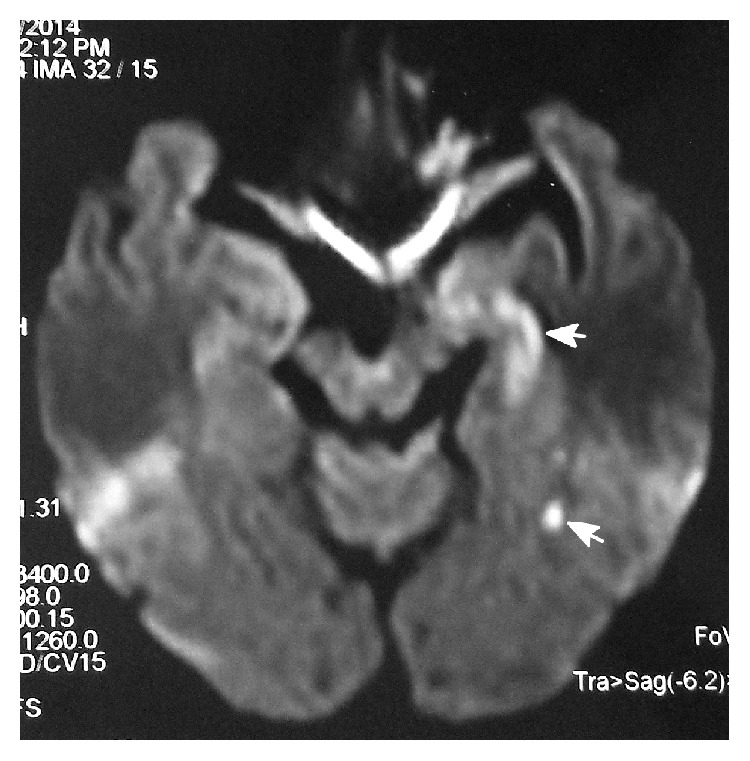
DWI of case 9 after TGA showing small acute infarctions (arrow) in left hippocampus and temporal lobe.
